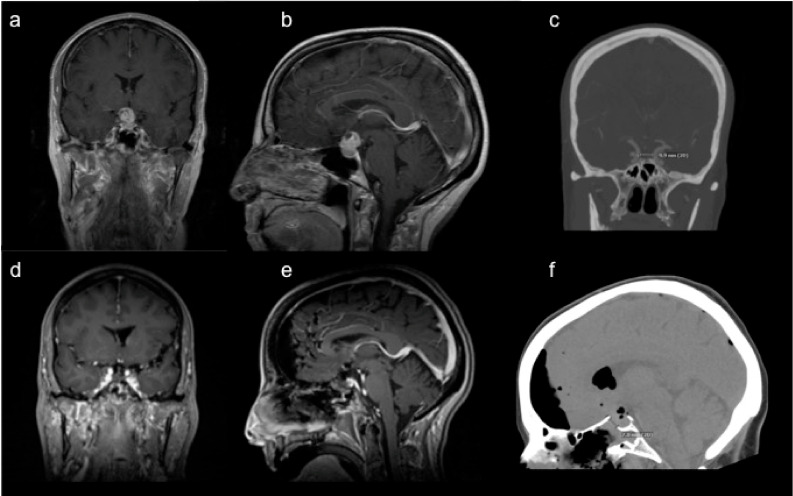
Relevant peri-operative imaging. A 67-year-old female presented with subjective decline in vision over several months and was diagnosed with bitemporal hemianopsia. Representative (a) coronal and (b) sagittal T1-weighted post-contrast magnetic resonance image (MRI) revealed a heterogeneously enhancing 1.7 cm sellar/suprasellar mass, consistent with a diagnosis of craniopharyngioma. (c) Pre-operative coronal computed tomography angiography (CTA) demonstrated an approximate 1 cm inter-carotid distance, relevant for surgical planning. Representative post-operative (d) coronal and (e) sagittal T1-weighted post-contrast MRI showed gross total resection through a (f) minimally invasive sub-centimeter craniotomy via an endoscopic endonasal transphenoidal approach, as shown on sagittal CT.
